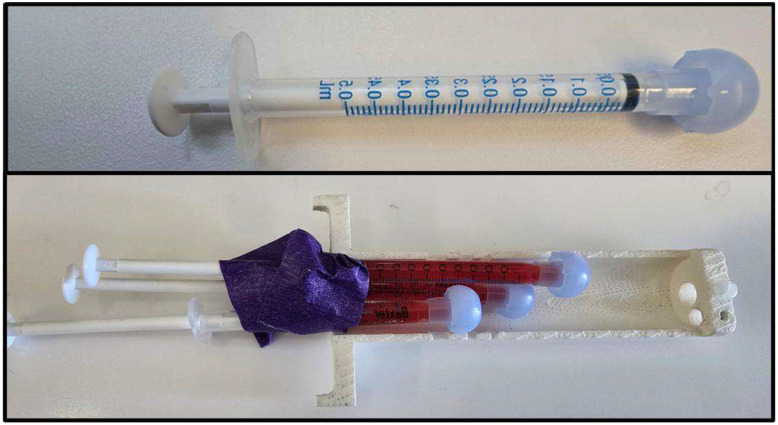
Unfilled 0.5 cc Baxter syringe (top), and three Baxter syringes filled with FDG solution (red dye) on the modified NCT (bottom).
